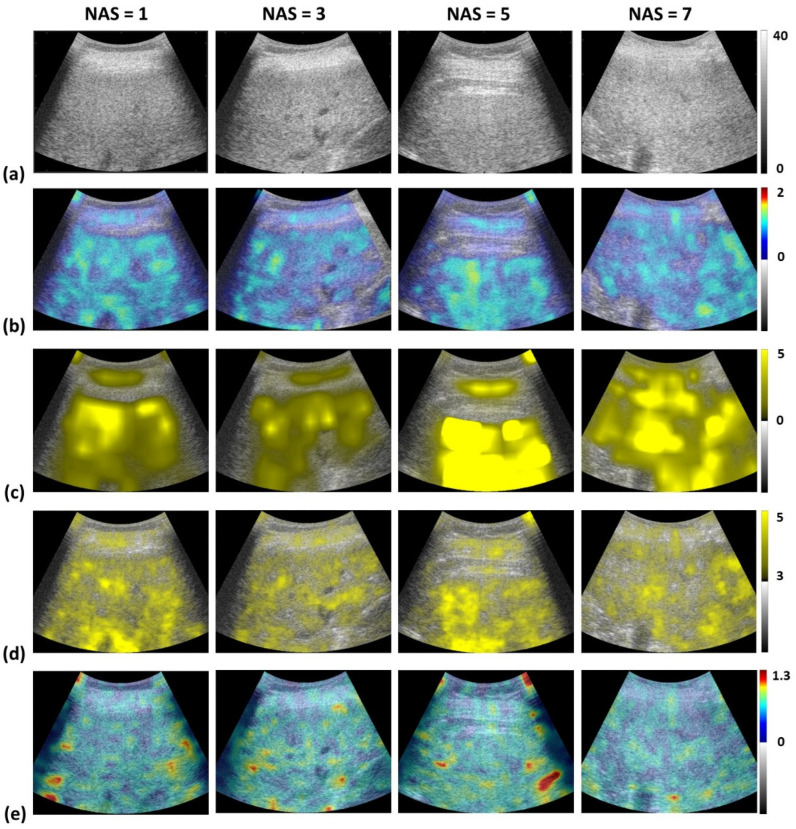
Representative (a) B-mode, (b) Nakagami, (c) HK, (d) entropy, and (e) attenuation images across different NAS levels. The QUS and attenuation images exhibited increased brightness with higher NAS, with visibly greater intensity in the NASH group compared to the non-NASH group. HK: homodyned-K; NAS: NAFLD activity score; QUS: quantitative ultrasound; NASH: nonalcoholic steatohepatitis.
